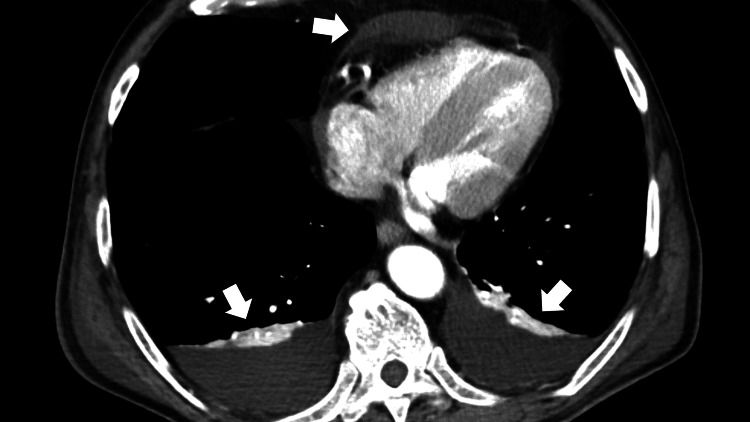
An enhanced abdominal computed tomography scan (transverse plane) showing generalized edema of the periarterial lesions around the superior and inferior mesenteric arteries (arrows).
